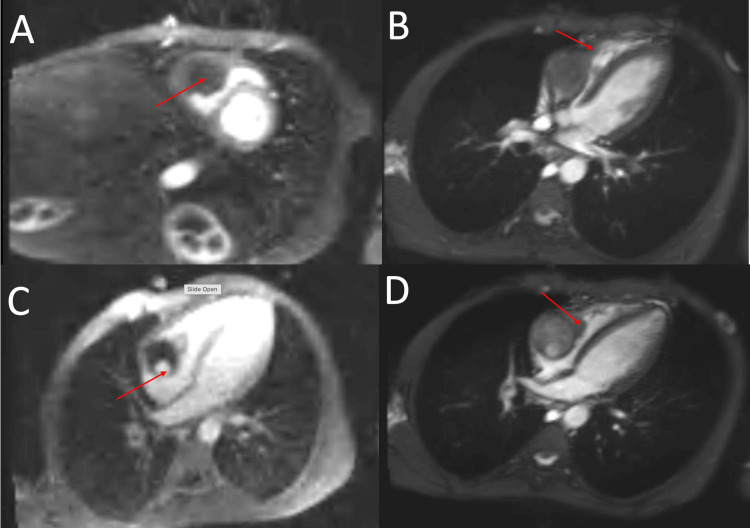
Cardiac MRI: Right atrial mass with features of malignant thrombus continuous with IVC thrombus. (A) Large atrial mass (arrow). (B) Partial prolapse into the right ventricle (arrow). (C) Heterogeneous right atrial mass with central hypointense thrombus and a small hyperintense viable component (arrow), prolapsing into the right ventricle. (D) Lobulated right atrial mass with irregular contours extending toward the tricuspid valve (arrow). Images were obtained from the authors’ institutional database. IVC, inferior vena cava
